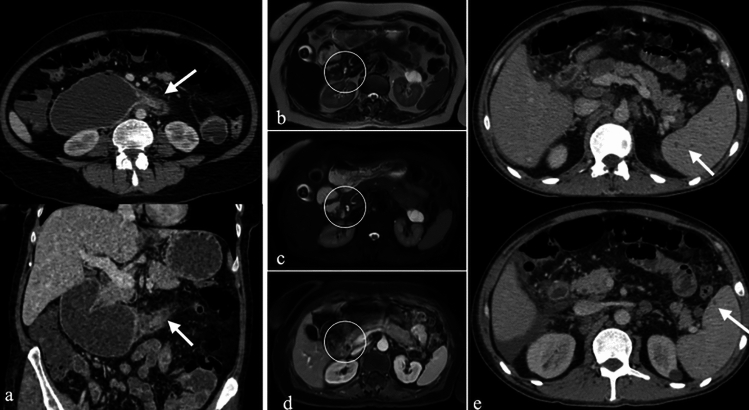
a. Axial and coronal IV contrast-enhanced CT images of a 42-year-old female patient with Crohn’s disease, showing short-segment stenosis, wall thickening, and submucosal fat deposition at the third (D3) segment of the duodenum (arrows), as well as aneurysmal dilatation of the second (D2) segment. MRI images b. T2-weighted, c. fat-saturated T2-weighted, and d. post-contrast fat-saturated T1-weighted) of a 51-year-old female patient with sarcoidosis, showing mucosal thickening and luminal narrowing (circles). e. IV contrast-enhanced CT images of a 68-year-old male patient with duodenal tuberculosis, revealing wall thickening, enhancement, and paraduodenal heterogeneity (arrows), along with intra-abdominal free fluid, splenomegaly, and multiple splenic tuberculous lesions
